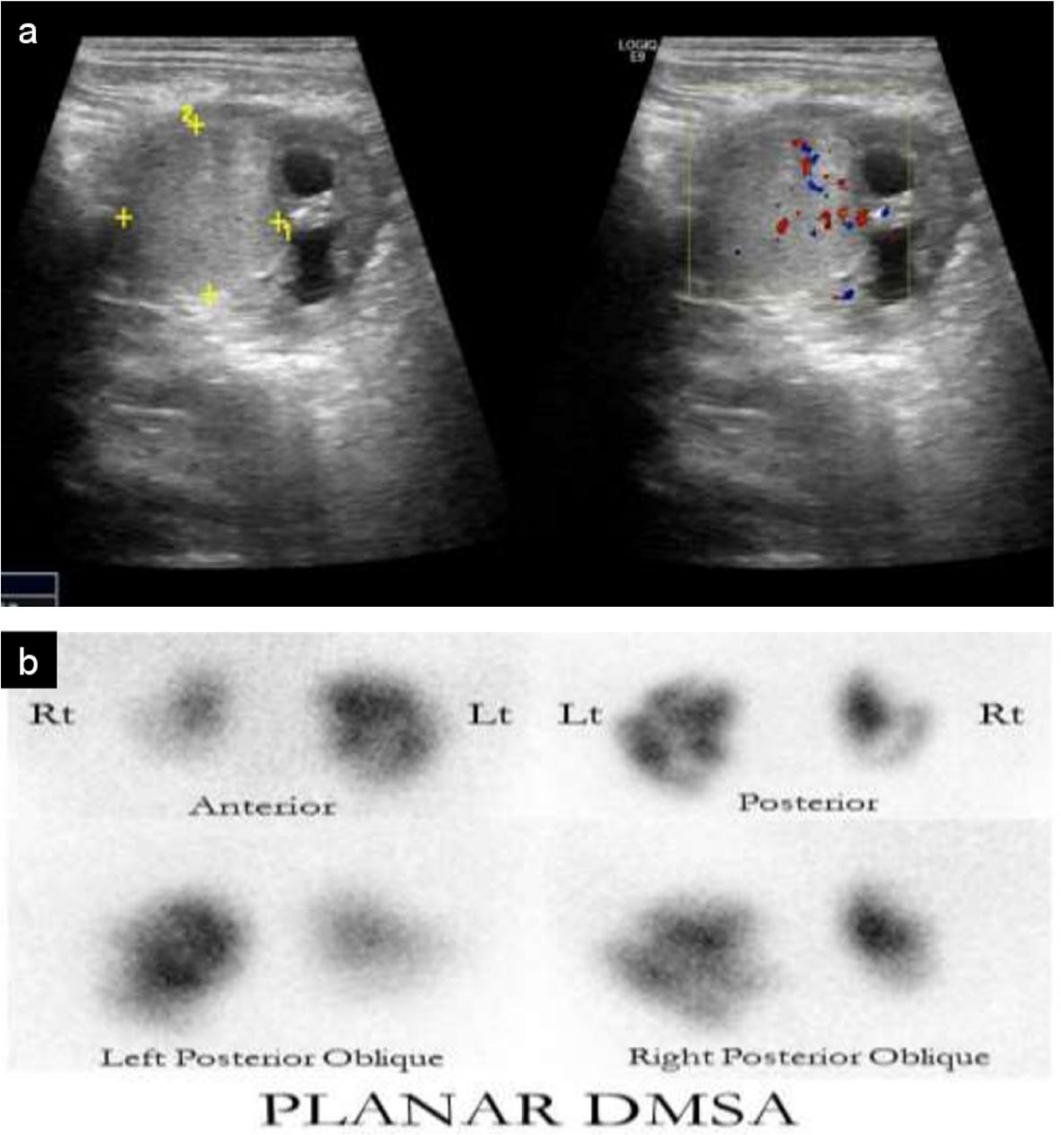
A US image shows a right renal mass. On color Doppler, the mass demonstrates internal vascularity. B DMSA scan images show multiple areas of scarring in both kidneys as well as nodular uptake in the right kidney most consistent with FPT (arrow)
